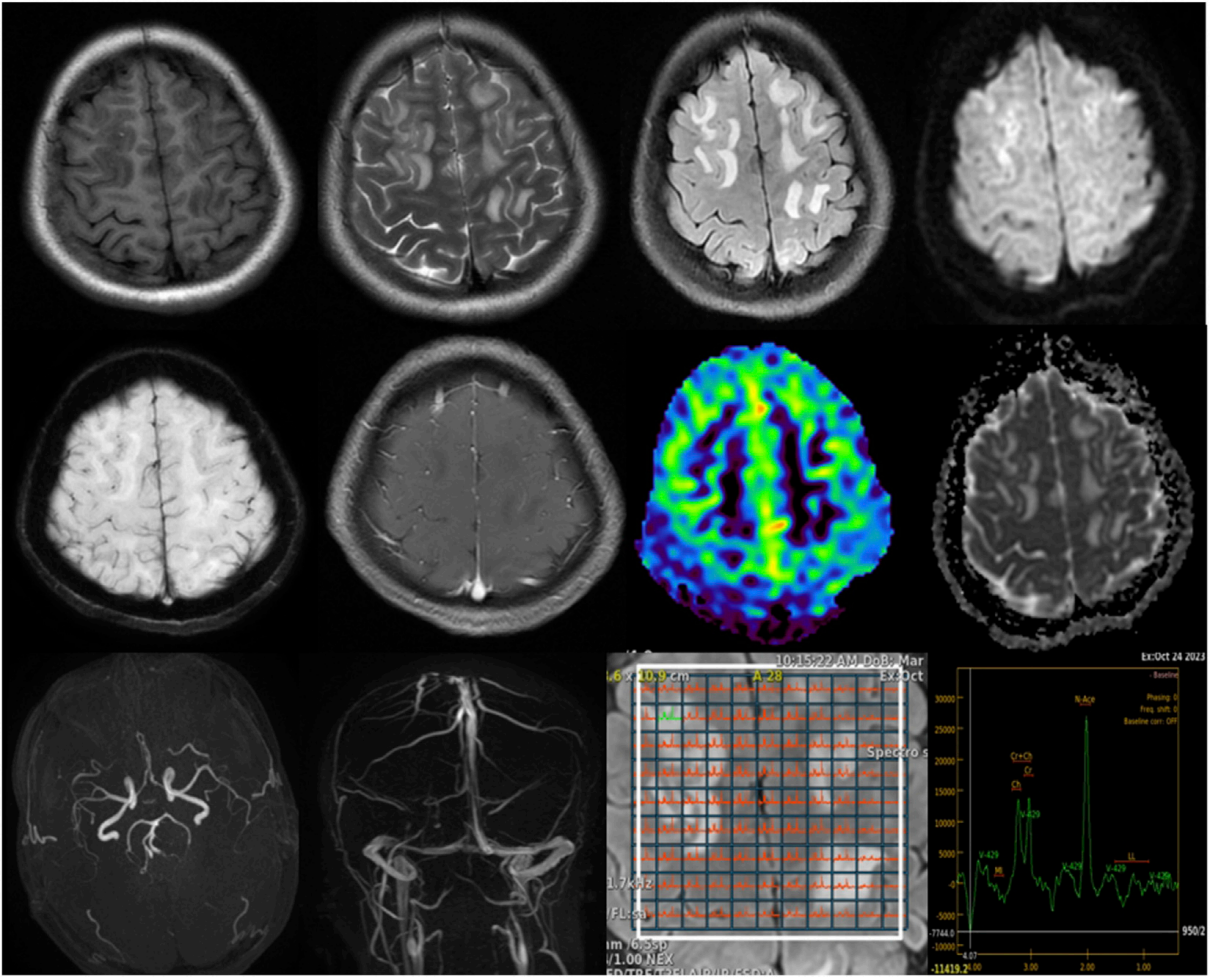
Brain MRI findings: T1-weighted images show slightly hypointense to isointense lesions, while T2-weighted and FLAIR sequences reveal hyperintense lesions predominantly involving the bilateral cerebral white matter with asymmetric distribution. DWI demonstrates high signal intensity, with corresponding high values on the apparent diffusion coefficient ADC map, indicating vasogenic edema. SWI shows no significant susceptibility artifacts. Post-contrast images reveal mild to moderate enhancement. MRS, MRA, and MRV findings are unremarkable, as shown.
